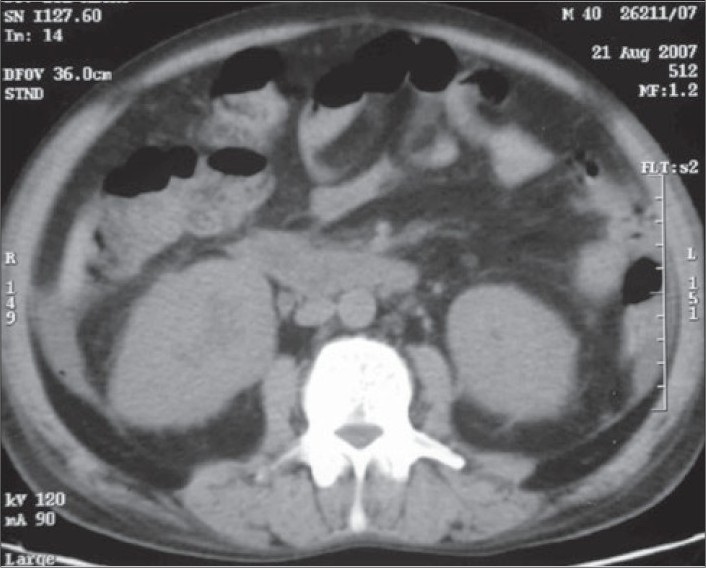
Axial CT sections at the level of the pelvis shows presence of ascites
